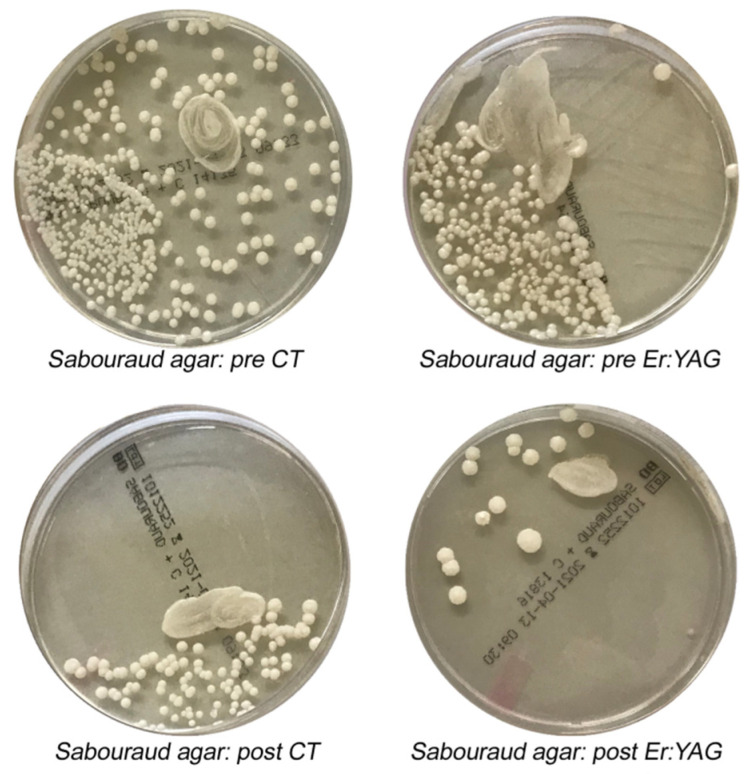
Difference in Candida albicans (*C. albicans*) colonies growth after Er:YAG treatments (right) vs. CT (left). Pre CT: pre-treatment with conventional therapy; post CT: post-treatment with conventional therapy; pre Er:YAG: pre-treatment with laser therapy; post Er:YAG: post-treatment with laser therapy.
